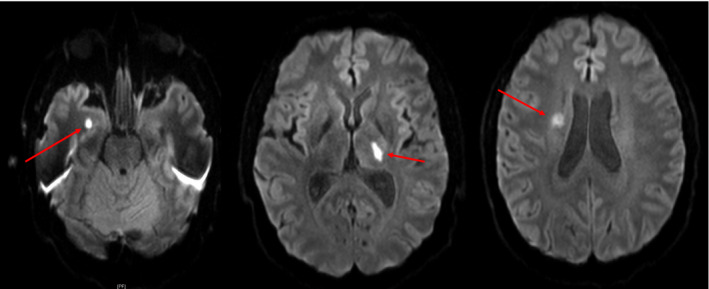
MRI brain without contrast, diffusion‐weighted sequence (DWI). There is restricted diffusion in the left thalamus and in the bilateral anterior temporal lobes that could represent acute infarcts. Late subacute to chronic infarct in the right corona radiata and the superior right basal ganglia.
